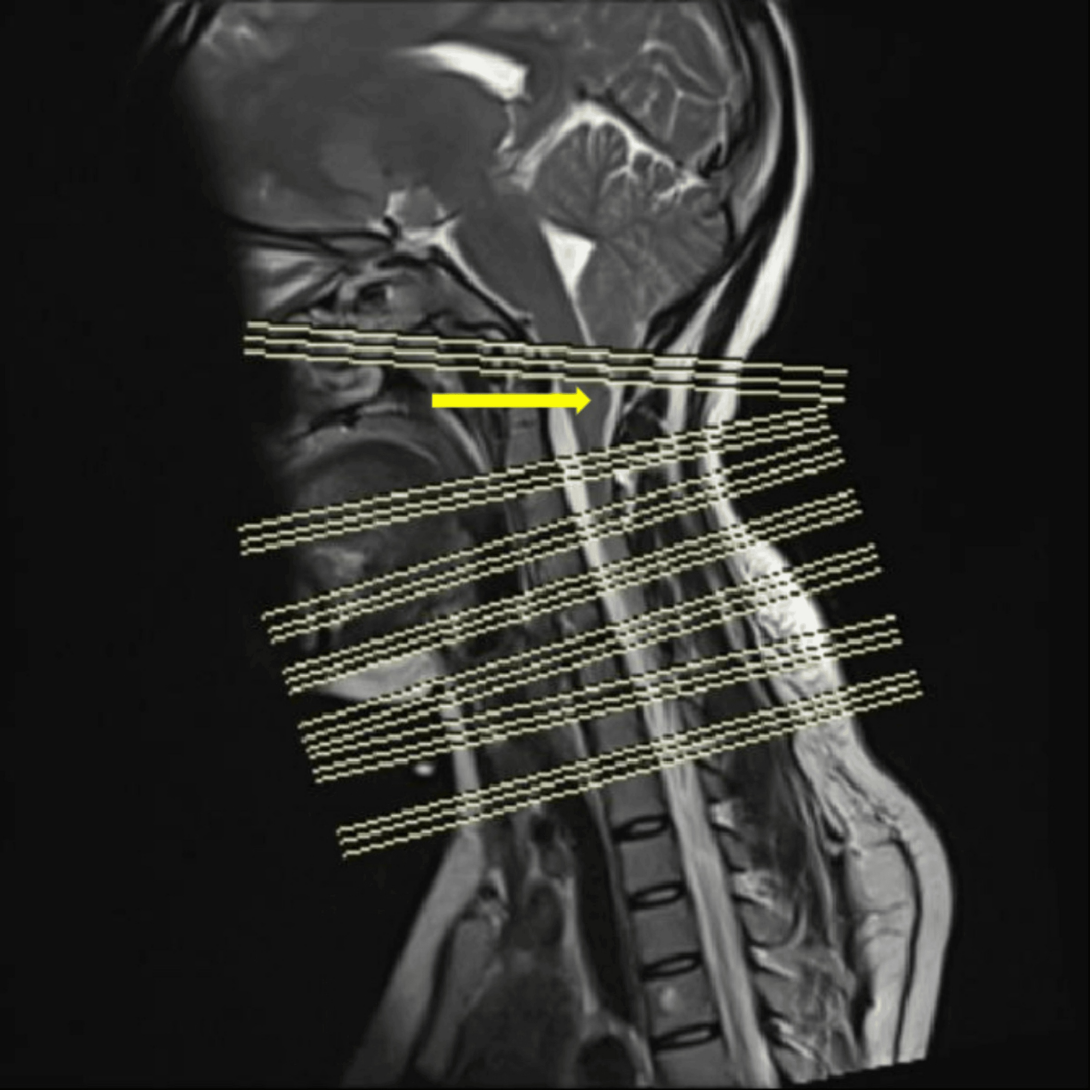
MRI spine T2 sagittal section cerebellar tonsils were found to be slightly displaced below the Mcrae line, suggesting Arnold Chiari malformation type I (Triple line demarcation is done here to represent the inter-vertebral disc space, with the first line being the Mcrae line)
